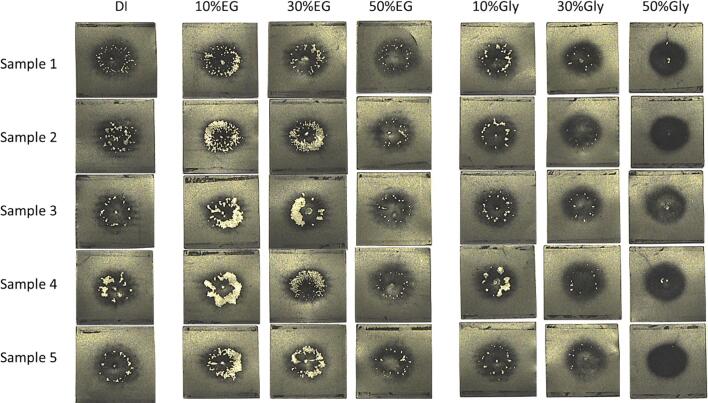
Comparison of ultrasonic delamination strength of different solutions on a 3 cm × 3 cm piece of LiB cathode. The delamination was performed using a 20 mm-Φ sonotrode for 10 s, at a sample-to-sonotrode distance of 5 mm, operating at an intensity of 119 W/cm^2^.
